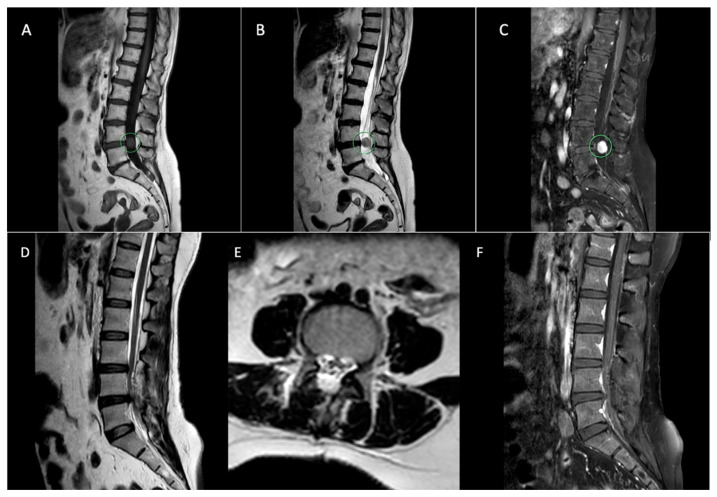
Preoperative and postoperative MRI findings of Case 1. Sagittal preoperative T1-, T2-, and contrast-enhanced T1-weighted images (A–C) demonstrate a well-circumscribed intradural extramedullary lesion at the L4–5 level, appearing isointense on both T1- and T2-weighted sequences with homogeneous contrast enhancement (circles). Postoperative sagittal and axial T2- and contrast-enhanced T1-weighted images (D–F) confirm gross total resection with no evidence of residual tumor.
